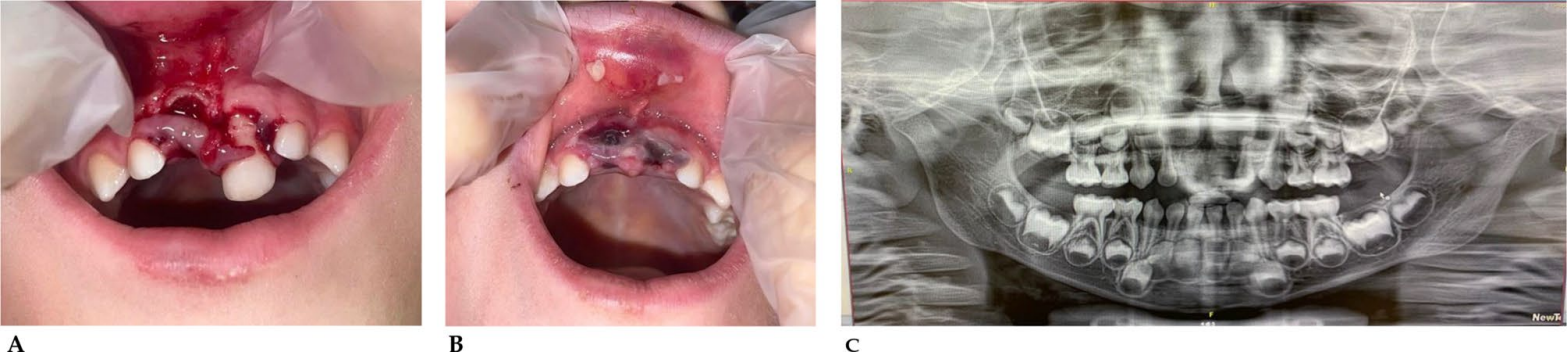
A: Loss of tooth 5.1 and dislocation of tooth 6.1. B: Fragments of tooth 5.1 in the upper lip. C: Panoramic x-ray of the patient’s dental arches
